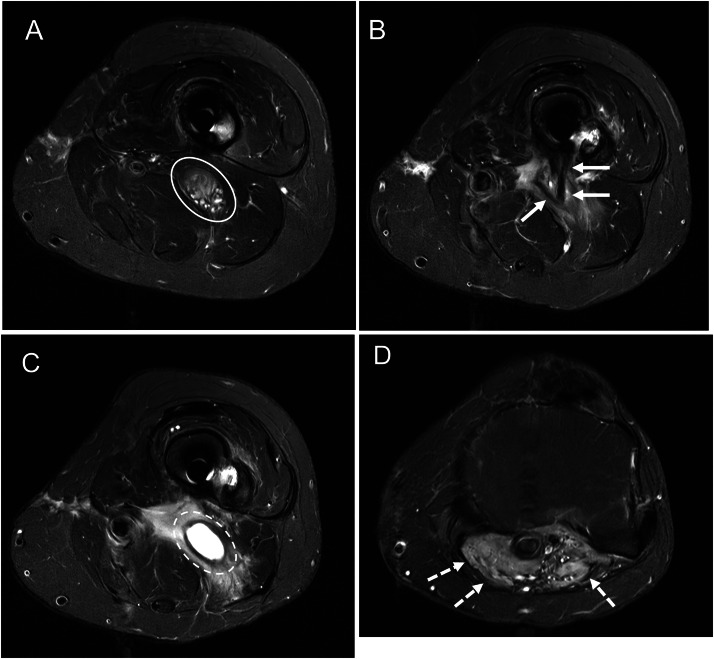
(A–C) Axial T2-weighted fat-saturated images of the distal femur show fusiform enlargement and fascicular expansion of the proximal stump of the transected sciatic nerve (white oval), consistent with traumatic neuroma. A posteriorly directed bone fragment with T2- hypointense cortical margins (arrows) transects the nerve just below this level. Immediately inferior to the bone fragment there is cystic dilation of the distal stump (dashed oval). (D) Axial T2-weighted fat-saturated image at the proximal tibia demonstrates diffuse edema within the gastrocnemius muscles (dashed arrows), indicative of denervation changes.
